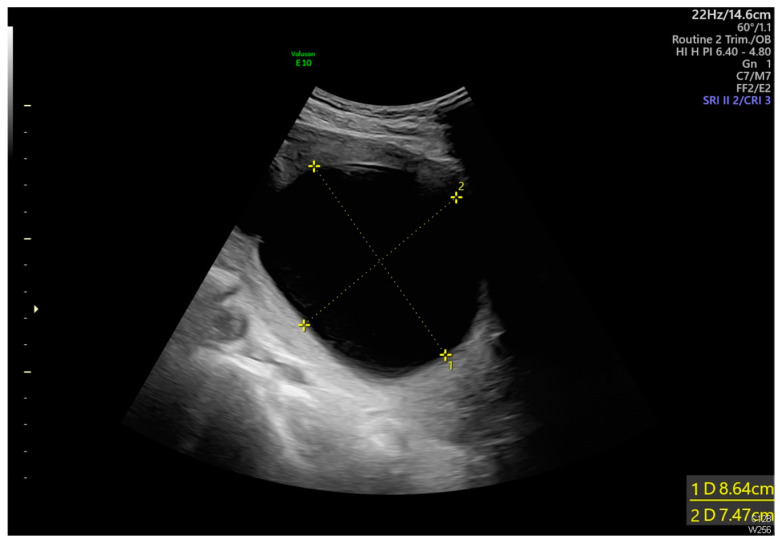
Transabdominal ultrasound revealed an adhesion-induced pseudocyst measuring 8.6 × 7.4 cm located between the endocervical inlet and uterine cavity in the lower uterine segment (As shown by the dotted line distance). The inlet was identified at the 10 o’clock position of the pseudocyst. The longest inlet of the adhesion-induced pseudocyst measured 2.6 cm (Figure 2). Uterine septa were present on anterior and posterior walls, resulting from intrauterine adhesions (Figure 3 and Figure 4). Transvaginal ultrasound showed cervical length of 3.29 cm without dilation, excluding cervical incompetence (CI) (Figure 5). Detailed second-trimester ultrasound detected no gross fetal anomalies or bulging uterine diverticulum. Fetal limbs and head moved freely into and out of the adhesion-induced pseudocavity without hyperechoic band-like lesions, making amniotic band syndrome unlikely. At 36 3/7 weeks’ gestation, cesarean delivery was performed due to pre-labor rupture of membranes and fetal breech presentation. Post-placental delivery examination revealed only a short fibrous tissue extending from the posterior uterine wall (Figure 6). The adhesion-induced septa may have been disrupted during fetal delivery. No adverse maternal or neonatal outcomes were observed.
